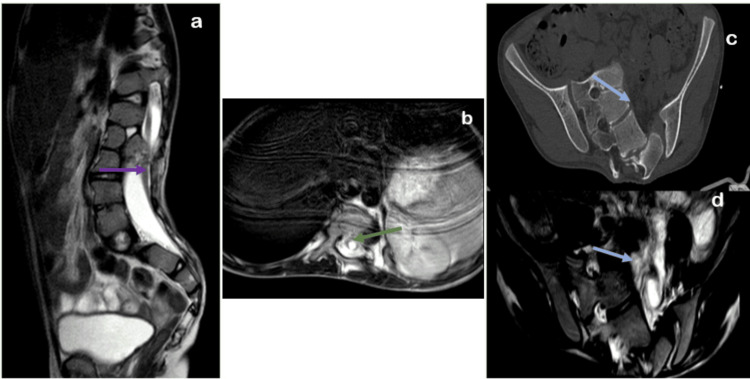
MRI spine T2 sequence: (a) sagittal image shows a low-lying tethered cord (violet arrow), (b) axial image shows syrinx/hydromyelia (green arrow). Axial section images from CT (c) and MRI T2 sequence (d) reveal partial unilateral left sacral agenesis (blue arrow).
